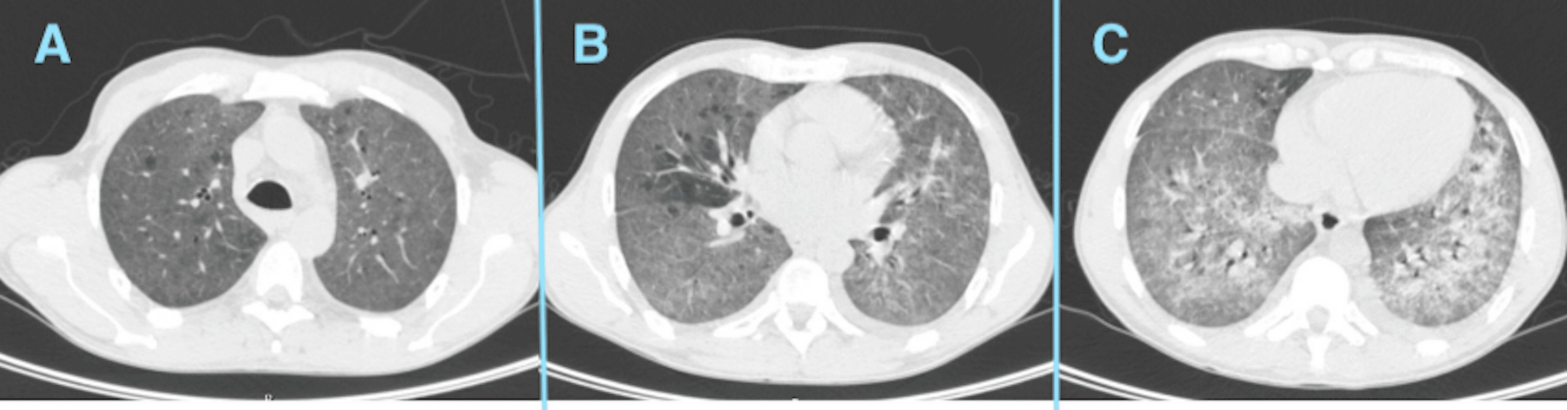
Bilateral ground-glass opacities on chest computed tomography at intensive care unit admission. (A) Axial CT image showing patchy bilateral ground-glass opacities predominantly in the upper lung fields, with preserved bronchovascular markings. (B) Progression of bilateral ground-glass opacities with increasing confluence and extension toward the perihilar regions. (C) More advanced bilateral ground-glass opacification with superimposed interlobular septal thickening, resulting in a “crazy-paving” appearance.
